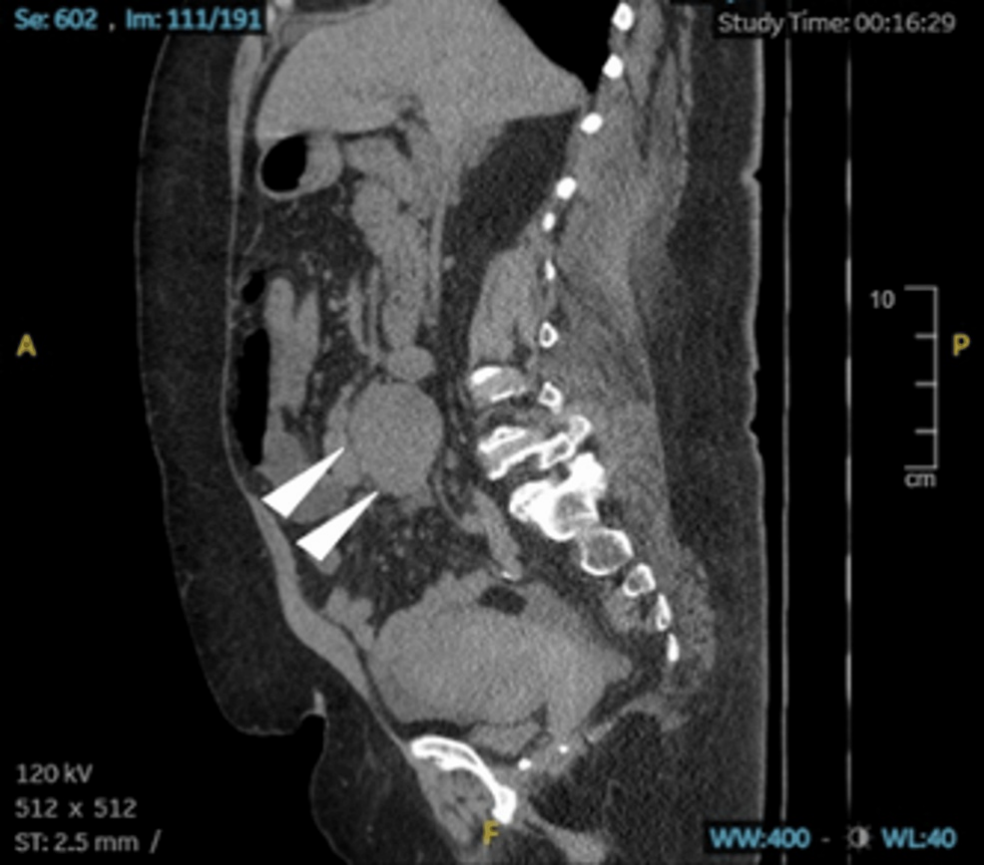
CT of the abdomen and pelvis without contrast, supine position, sagittal view displaying distended loops of small bowel, and adhesions near the NET (arrowheads) taken at initial ER presentation. NET: neuroendocrine tumor
